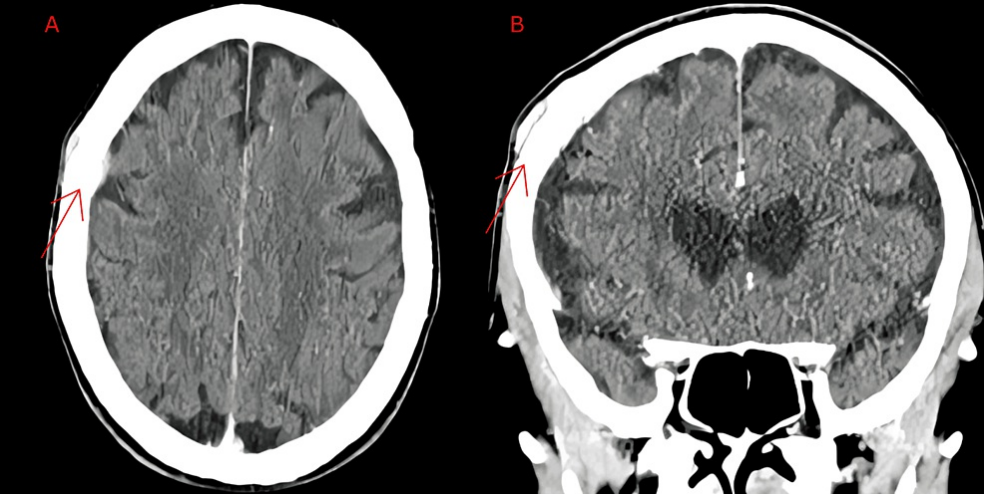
Computed tomography of the head showing an intracranial dural-based enhancing mass (red arrow) measuring 2.8 cm, which appears to extend through frontoparietal calvarium with a scalp component of similar size: (A) axial and (B) coronal views.
